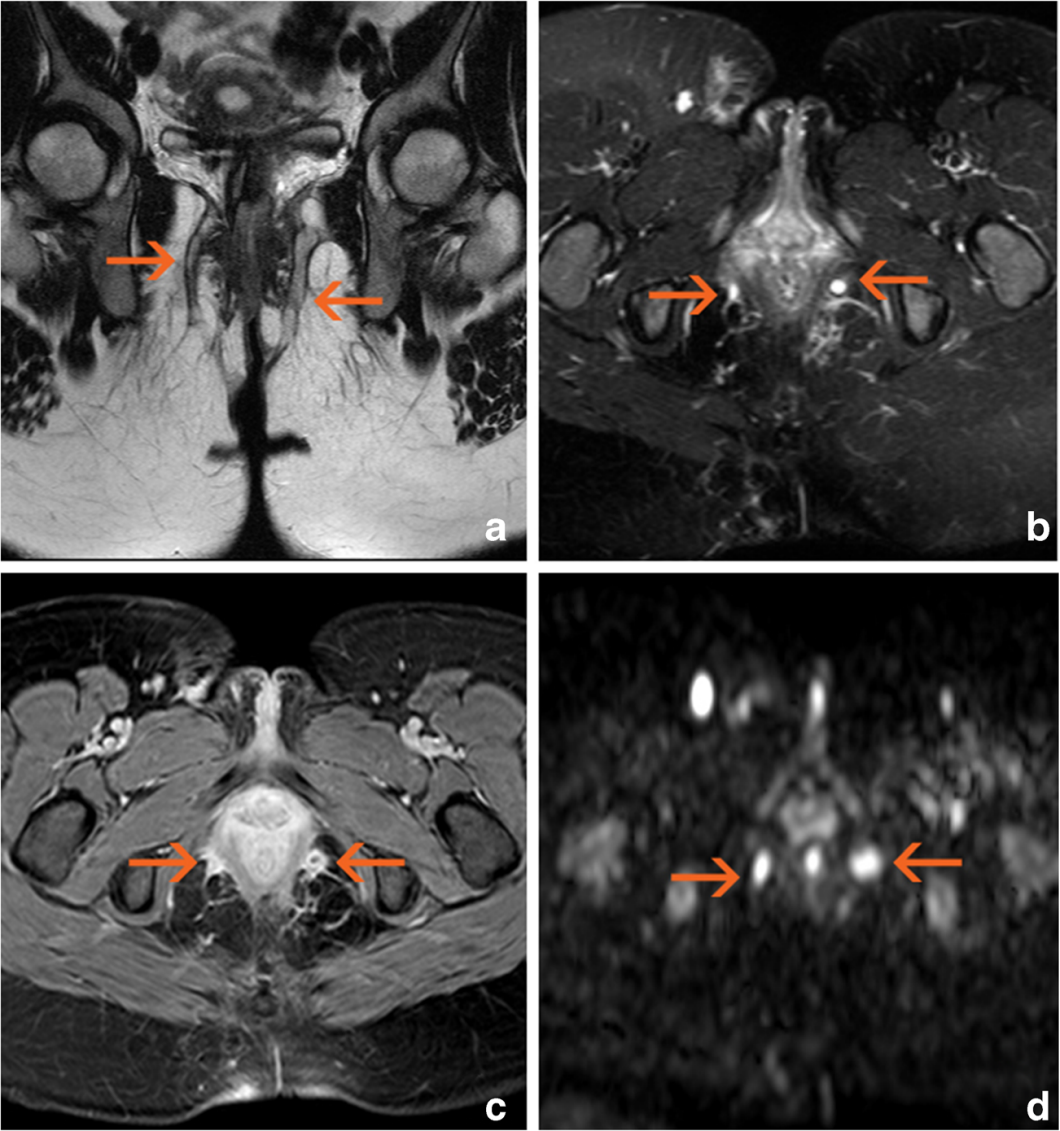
Complex bilateral transsphinteric fistula cursing across both ischioanal fossae (arrows). a Oblique-coronal T2-weighted image. b Oblique-transverse fat-supressed T2-weighted image. c Post-gadolinium oblique-transverse fat-supressed gradient echo T1-weighted image. d Native oblique-transverse native diffusion-weighted image with 800 s/mm^2^ b factor
